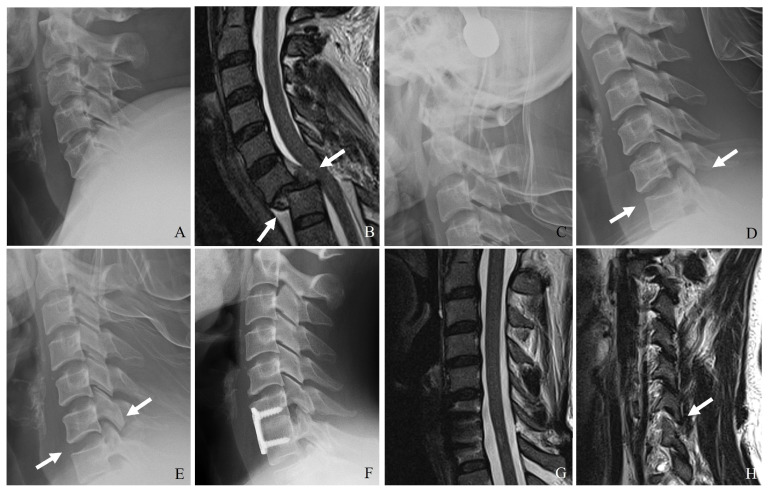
Plain X-ray (A) and magnetic resonance imaging (B) demonstrate bilateral facet dislocation and traumatic disc herniation at C6–C7 (white arrows). Successful closed reduction with skull traction was achieved (white arrows) (C–E). The patient subsequently underwent anterior cervical discectomy, open reduction, and fusion of C6–C7 (white arrow) (F–H).
